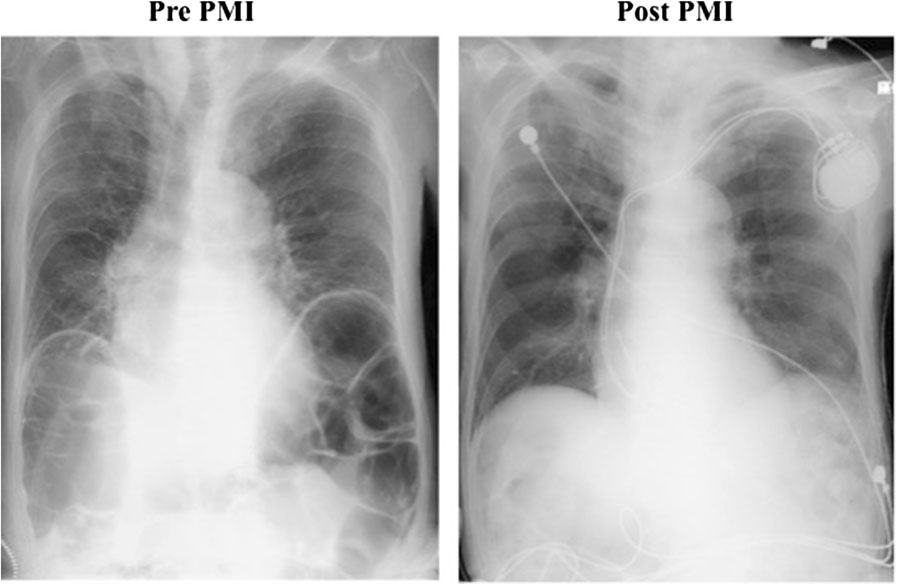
Chest X-ray examinations pre-PMI demonstrated significant dilatation of the bowels; however, this dilatation was improved after PMI with right ventricular apical pacing. *PMI* pacemaker implantation
